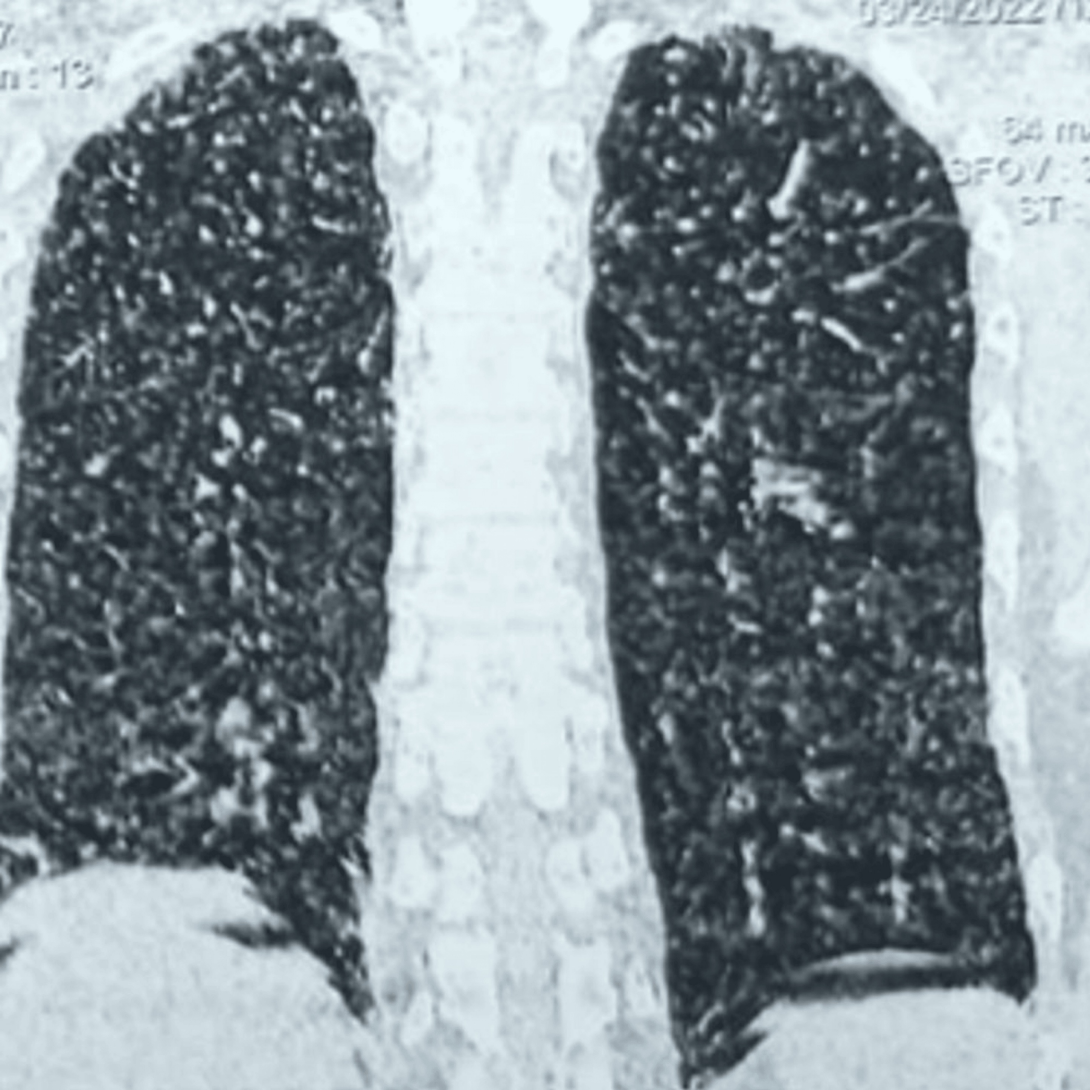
A coronal computed tomography of the lung window reveals opacity of size 1 to 3 mm all over the bilateral lungs (CT taken 12th day after X-ray)
